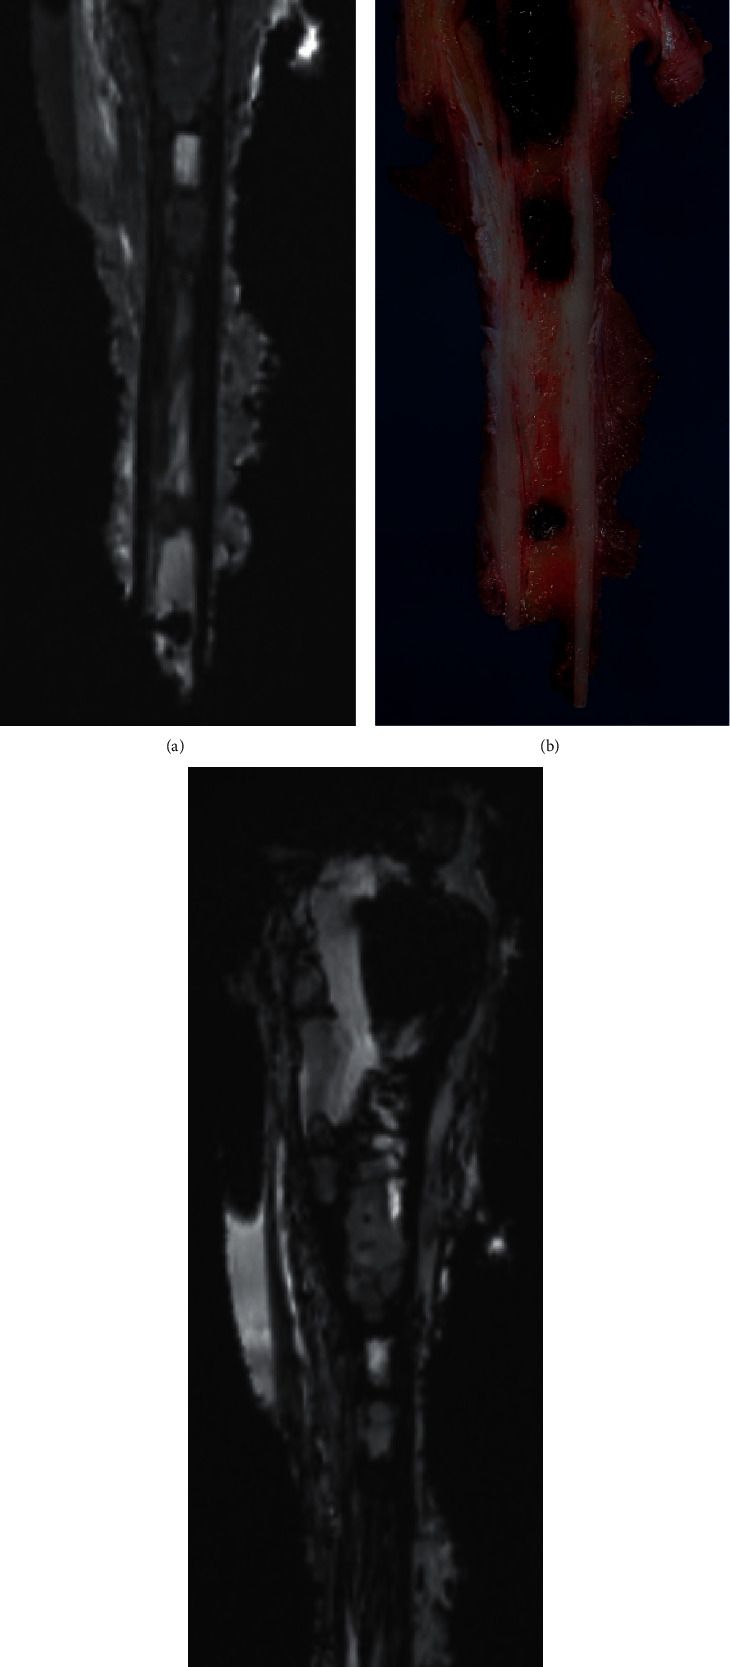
A 9-year-old girl with osteosarcoma of the distal radius. A step-cut was performed proximally for the stability of surgical reconstruction by using a PSI. Two margins were measured (medial and lateral part of the step-cut). Note the difficulty to visualize the cortical bone in the step-cut with MRI. (a) T1-weighted MRI; (b) macroscopic cut; (c) T2-weighted MRI.
